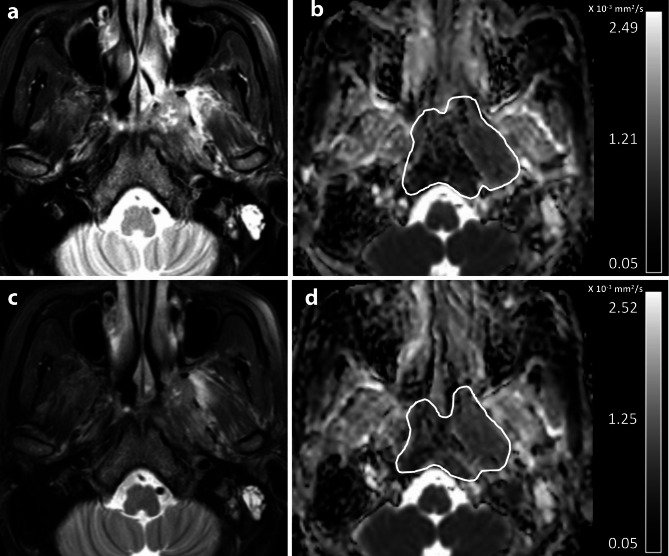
Pre-treatment (a-b) and post-IC (c-d) MRIs of a patient with stage T3 NPC that had recurrence 28 months after treatment. The axial images comprise T2-weighted fat-suppressed images (a and c) and ADC maps (b and d). The mean ADC_pre_ and ADC_post−IC_ extracted from the primary NPC (white contours) on the ADC maps were 0.89 × 10^− 3^ mm^2^/s, and 0.99 × 10^− 3^ mm^2^/s, respectively and the ΔADC% was 11.2%. The poor long-term outcome was predicted by a low percentage increase in mean ADC value (ΔADC% of < 34.2%) on the post-IC DWI compared with that on the pre-treatment DWI.
